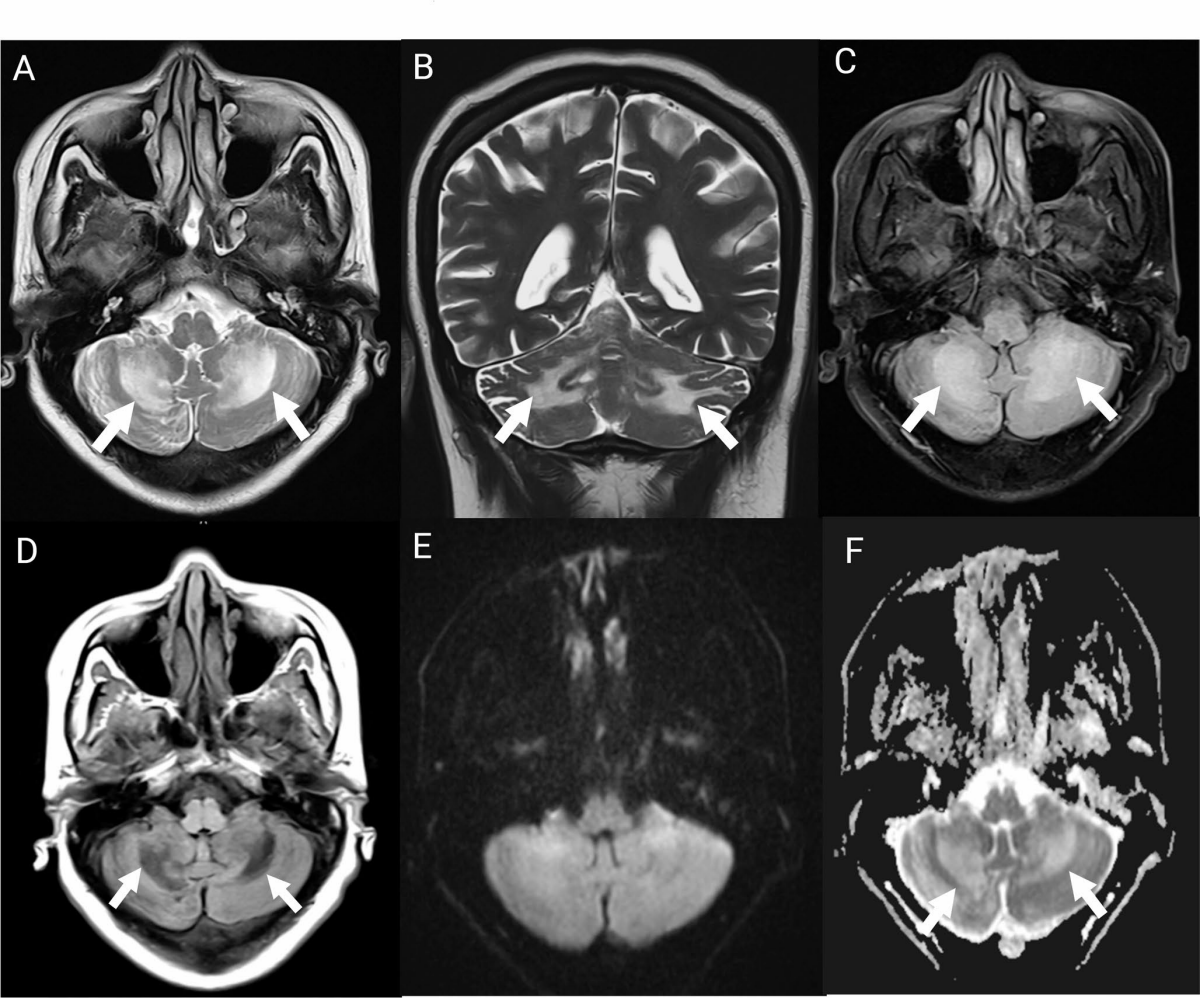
Magnetic resonance imaging of the brain. (A, B) Axial and coronal T2-weighted images show symmetrical hyperintensities involving the bilateral middle cerebellar peduncles and adjacent cerebellar white matter (white arrows). (C) Axial FLAIR image reveals similar hyperintensities. (D) Axial T1-weighted image demonstrates corresponding hypointensities. (E) Diffusion-weighted imaging (b = 1000 s/mm²) does not show restricted diffusion. (F) The apparent diffusion coefficient (ADC) map shows hyperintensity in the same regions, suggesting the T2 shine-through effect and excluding true diffusion restriction
